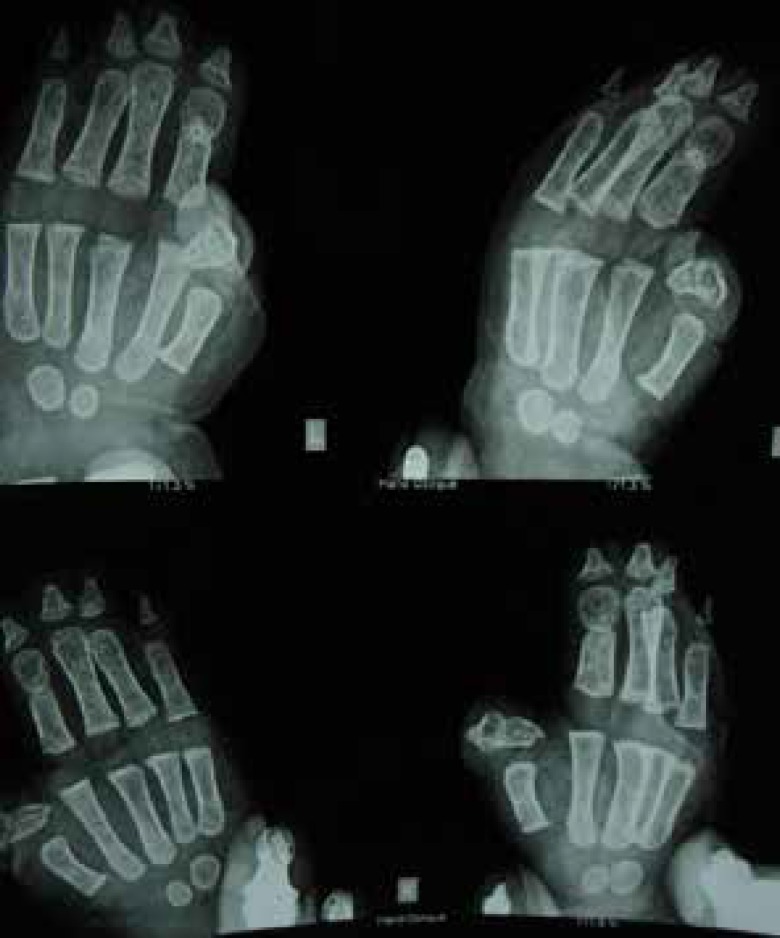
Digital x-ray of both hands (AP and oblique views).
